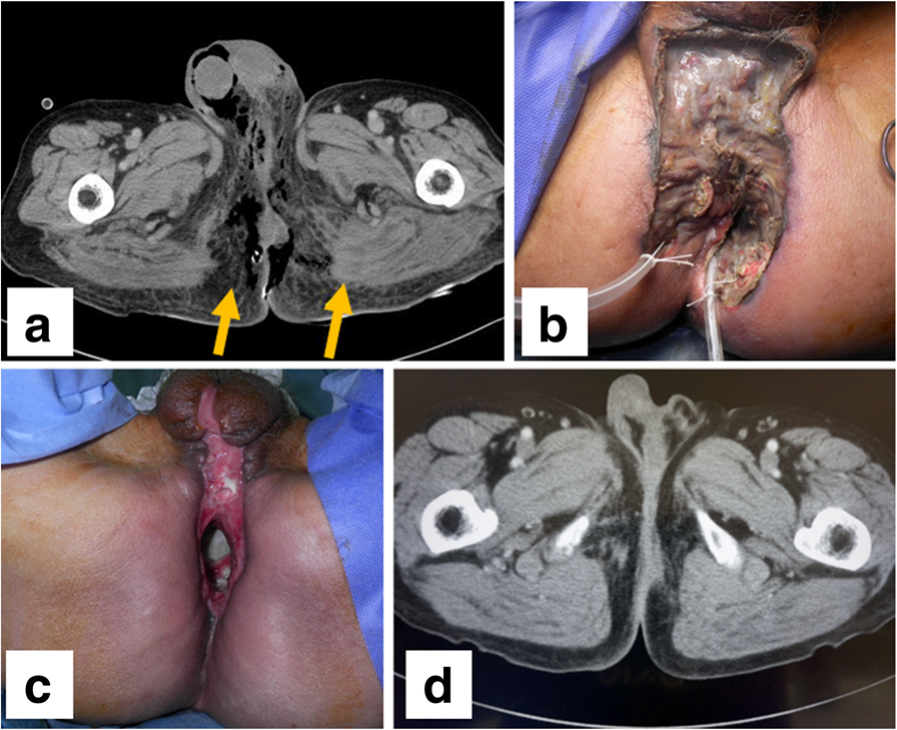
Computed tomography and pictures of the perineum and buttocks region. Computed tomography showed necrotizing soft tissue infection with large amounts of gas throughout the perineum (*arrows*) (a). The necrotic soft tissue in the perineum and buttocks surrounding rectal cancer was resected (b). The perineum and buttocks region on day 35 after total pelvic exenteration (c). Computed tomography 8 months postoperatively (d)
